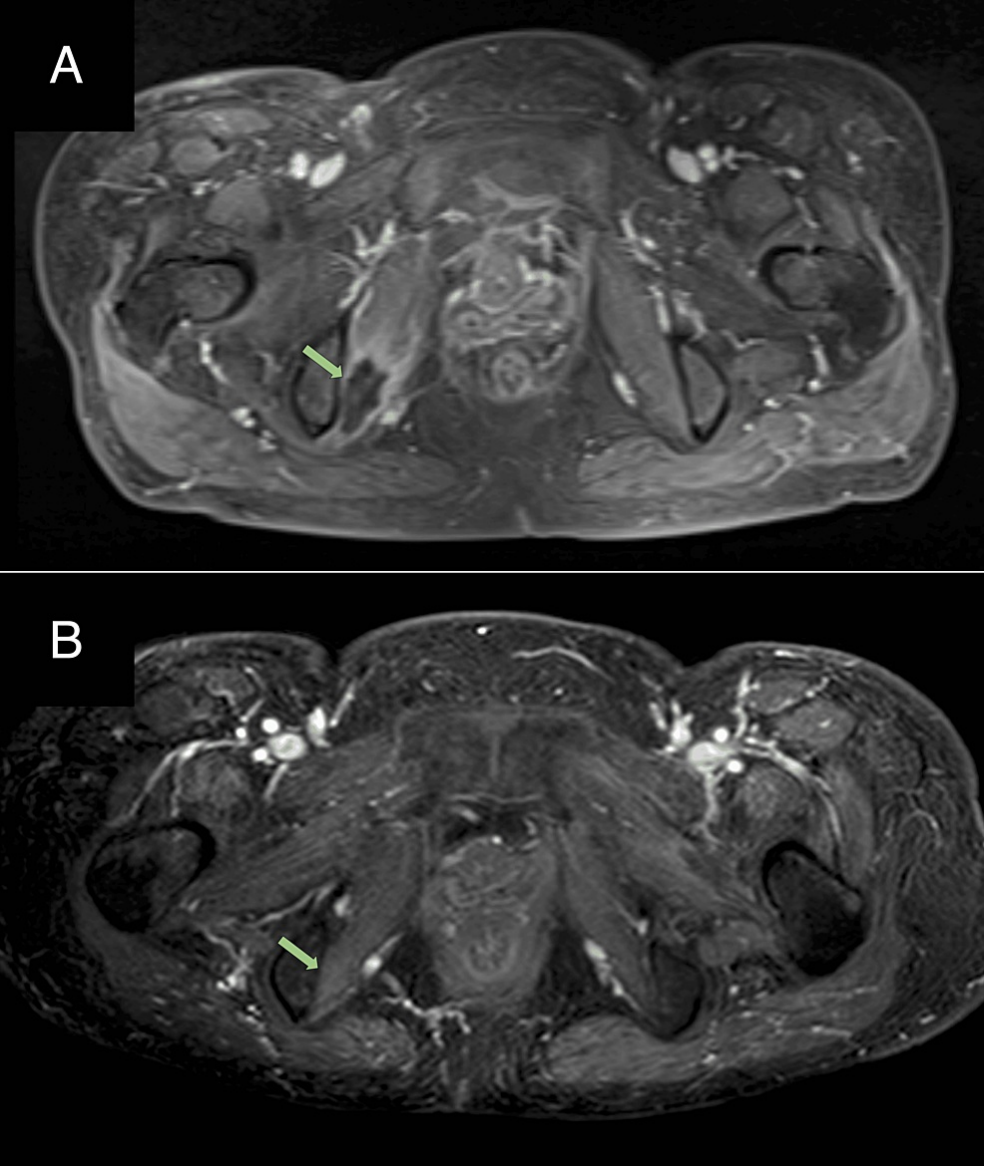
Contrast-enhanced MRI axial image before the second course of hyperbaric oxygen therapy (A) and after the treatment (B) (A) Contrast-enhanced MRI showed poor contrast mass in the right obturator internus muscle (green arrow). (B) The mass was resolved after the second hyperbaric oxygen therapy. MRI: magnetic resonance imaging
